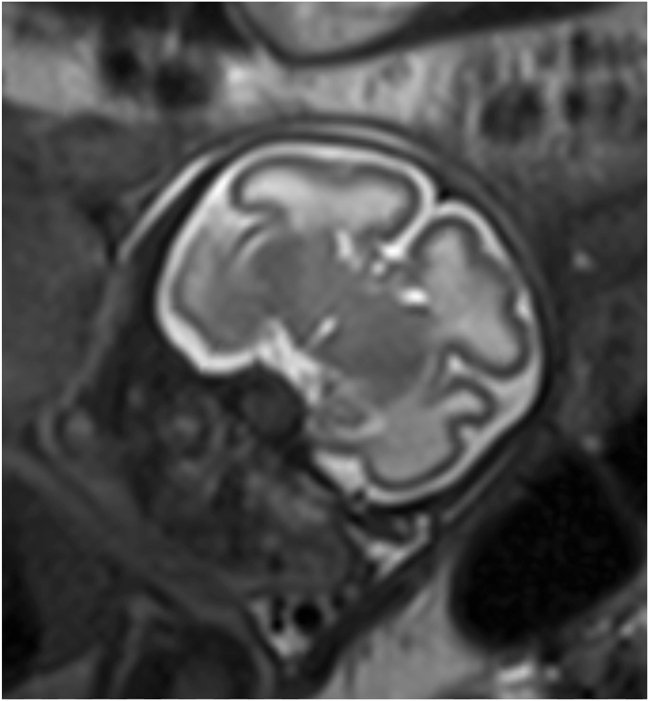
Fetal MRI at 33 + 2 weeks’ gestation showing a coronal plane at the position of the Sylvian fissure. Measurement of the sylvian fissure angle can help to identify cortical malformation. In our case, the gyrification at 34 weeks of gestation corresponded to that at 29 weeks.
